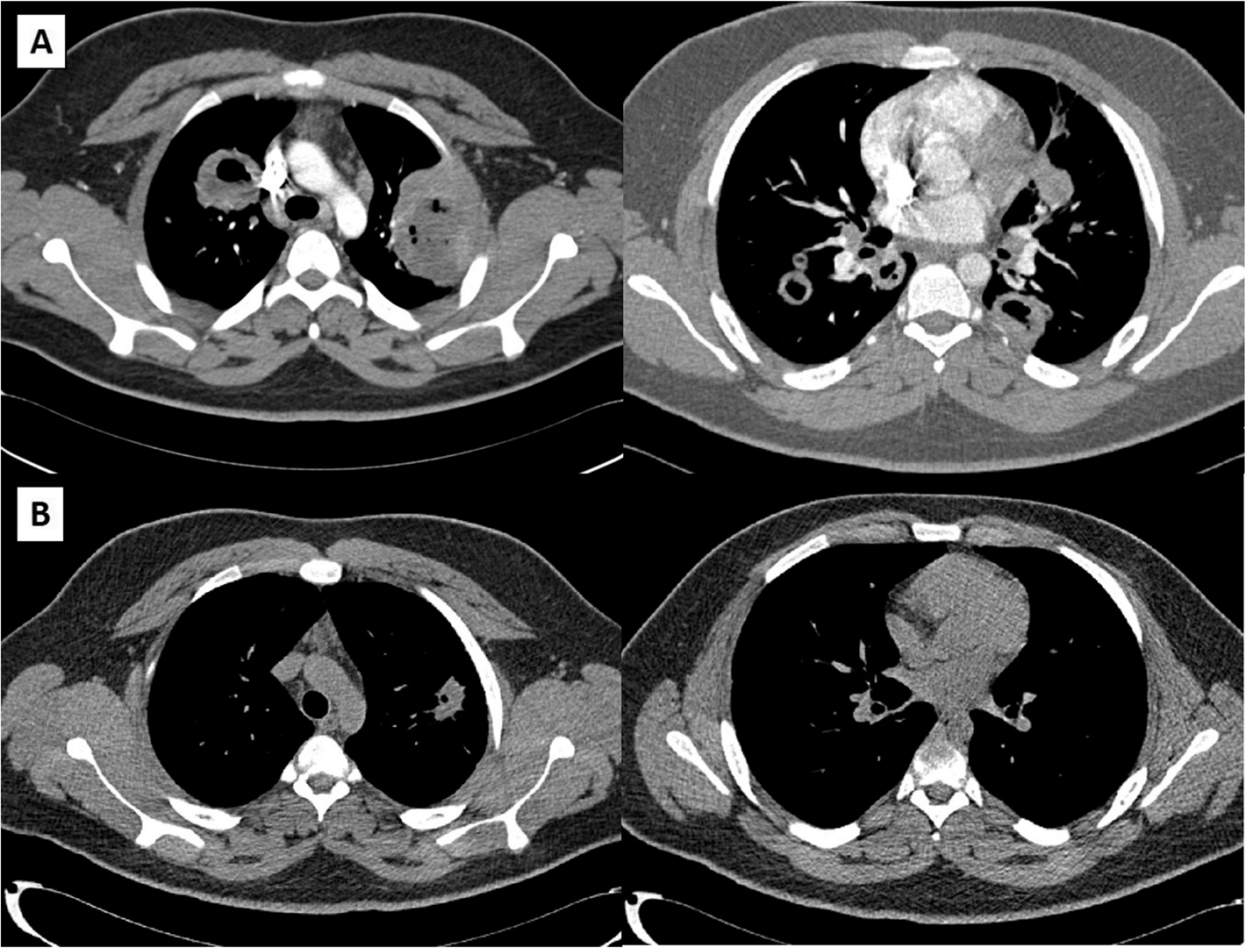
Chest CT findings at presentation (A) and 6-month follow-up (B)
